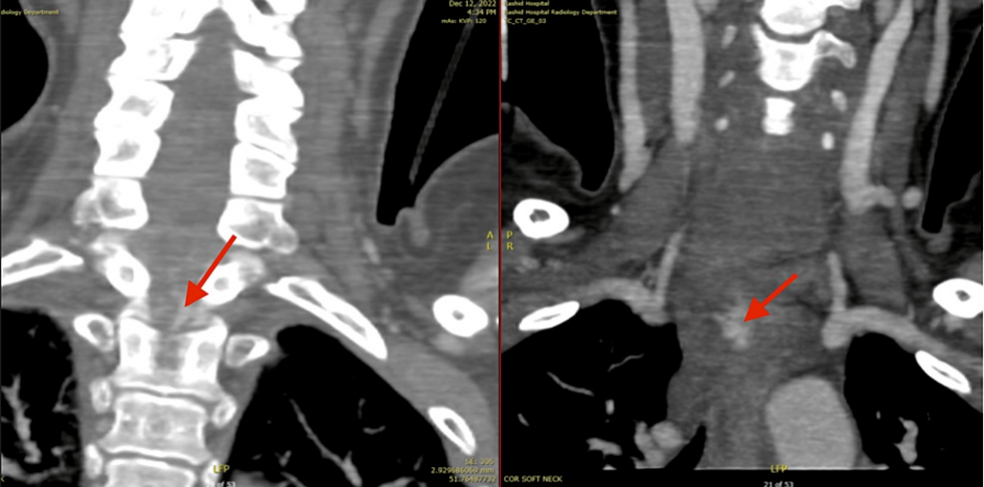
Coronal soft tissue CT windows reveal a large prevertebral and intraspinal haematoma from level C5-T2, with specks of contrast extravasation (Red arrow).
